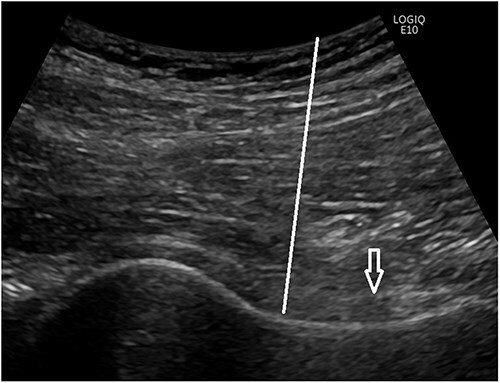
Ultrasound picture of the hip joint. The capsule of the hip joint is marked by an open arrow and the puncture line for Botox injection is marked with a line.
